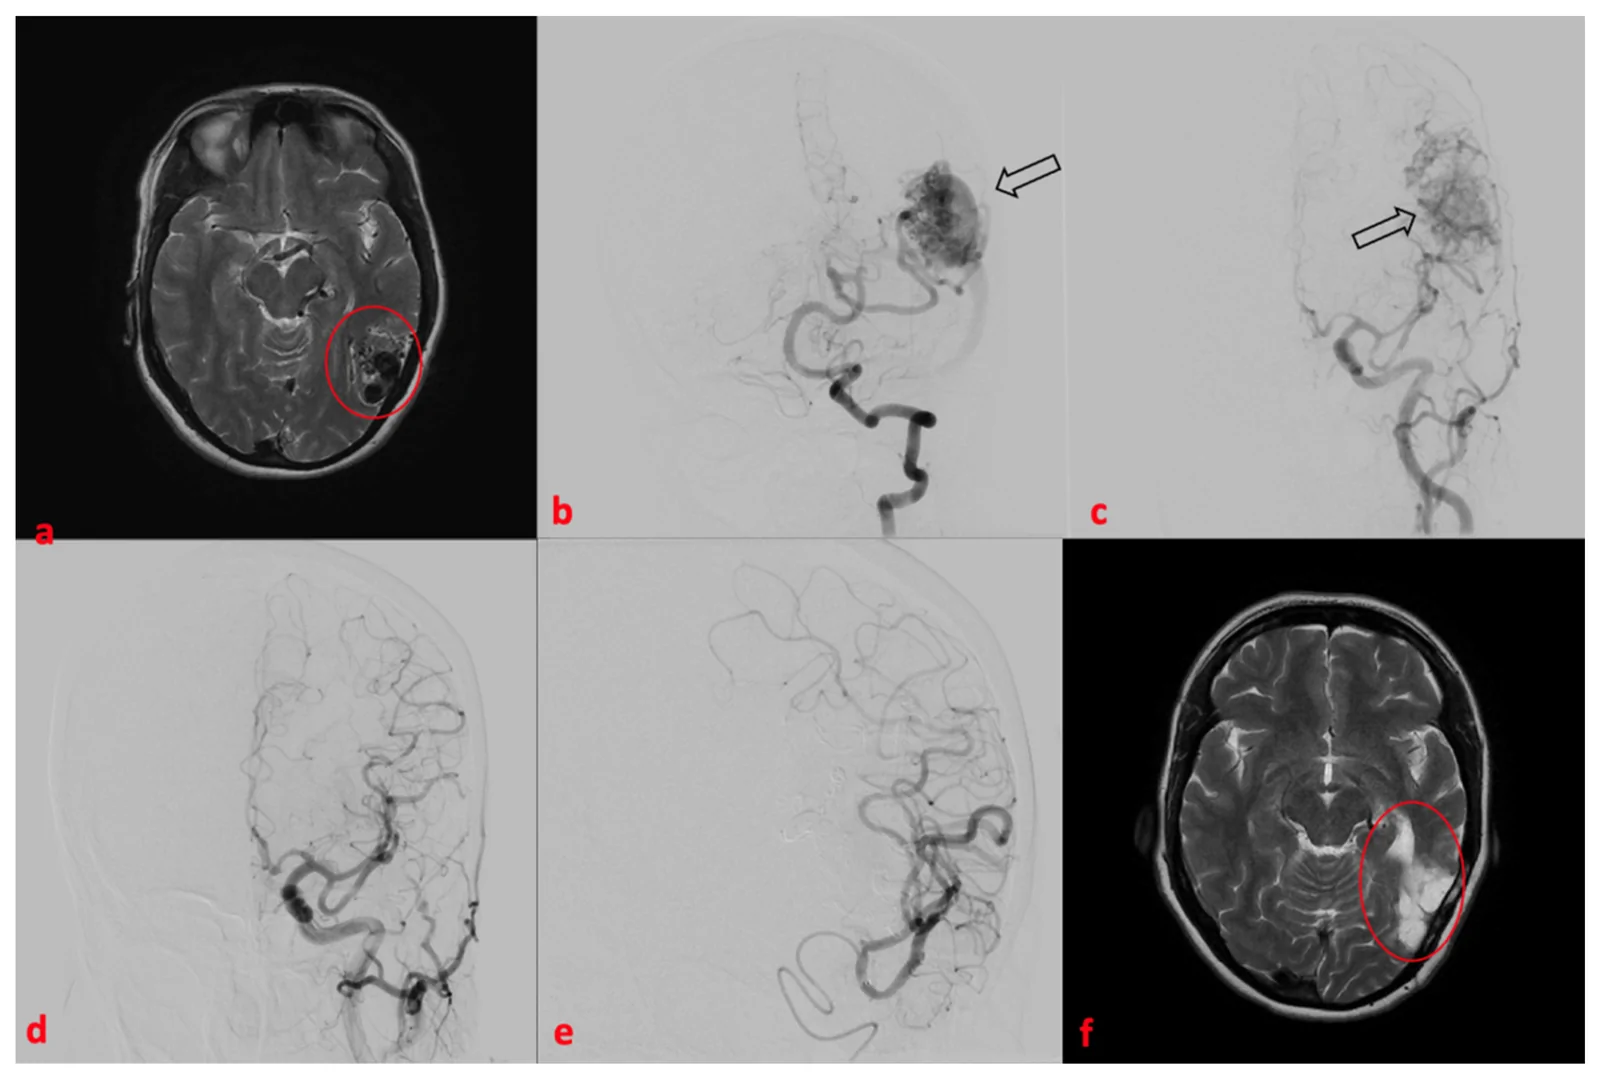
Endovascular-only case demonstrating pre- and post-treatment angiographic findings. Staged endovascular treatment of a left temporo-occipital arteriovenous malformation in a patient managed without same-session surgery. (a) Pre-treatment axial T2-weighted MRI at the level of the lateral ventricles. Serpiginous flow voids of the nidus are seen in the left temporo-occipital region (red circle), without mass effect, midline shift or hemorrhage. (b) Pre-treatment DSA, left vertebral artery injection, lateral projection, arterial phase. The compact nidus (open arrow) is supplied by posterior cerebral artery branches, with early venous filling in the arterial phase. (c) Pre-treatment DSA, left internal carotid artery injection, anteroposterior projection, arterial phase. Additional supply to the same nidus from the anterior circulation (open arrow) establishes dual arterial supply. (d,e) DSA after completion of staged embolization, left internal carotid (d) and left vertebral (e) injections. The anterior circulation supply shown in (c) and the nidus opacified in (b) no longer fill, and no residual shunt or early draining vein is identified from either territory. Normal posterior circulation branches remain patent. (f) Follow-up axial T2-weighted MRI one year after treatment, at a level comparable to (a). The previous flow voids (red circle) are replaced by high T2 signal with volume loss at the site of the treated malformation.
